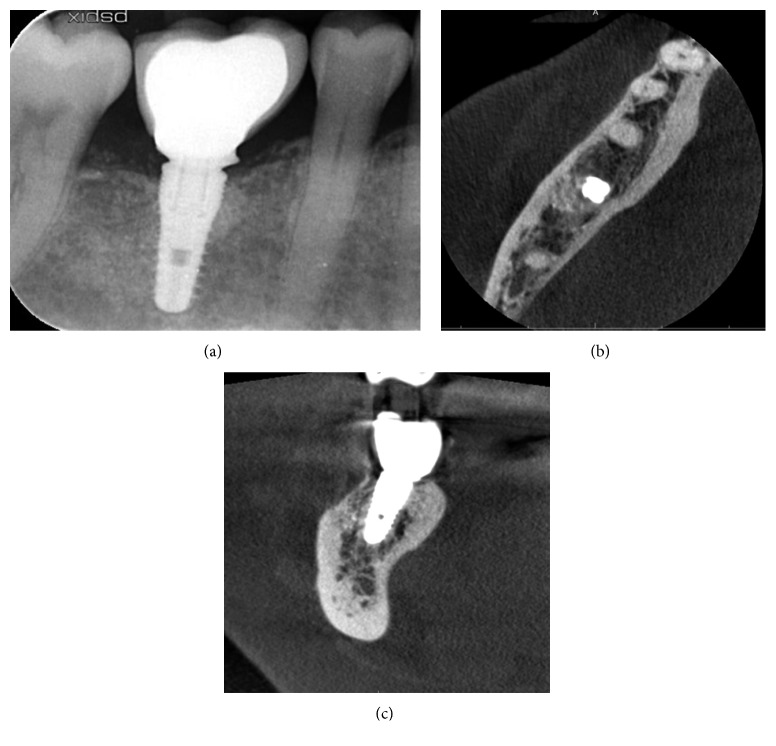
Radiological examination two years after loading of the implant: periapical X-ray and axial and coronal planes of the CBCT showing the preservation of the dimensions of the regenerated bone.
